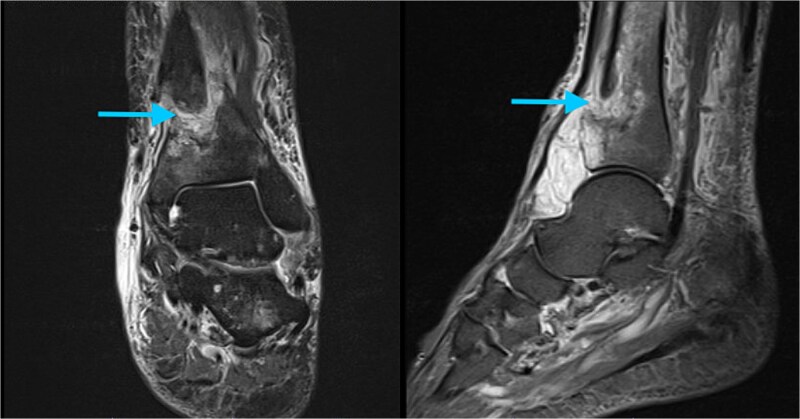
Preoperative MRI of the left ankle. Axial T2-weighted images demonstrate a well-circumscribed, hyperintense soft tissue lesion adjacent to the anterior cortex of the distal tibia (arrow).
